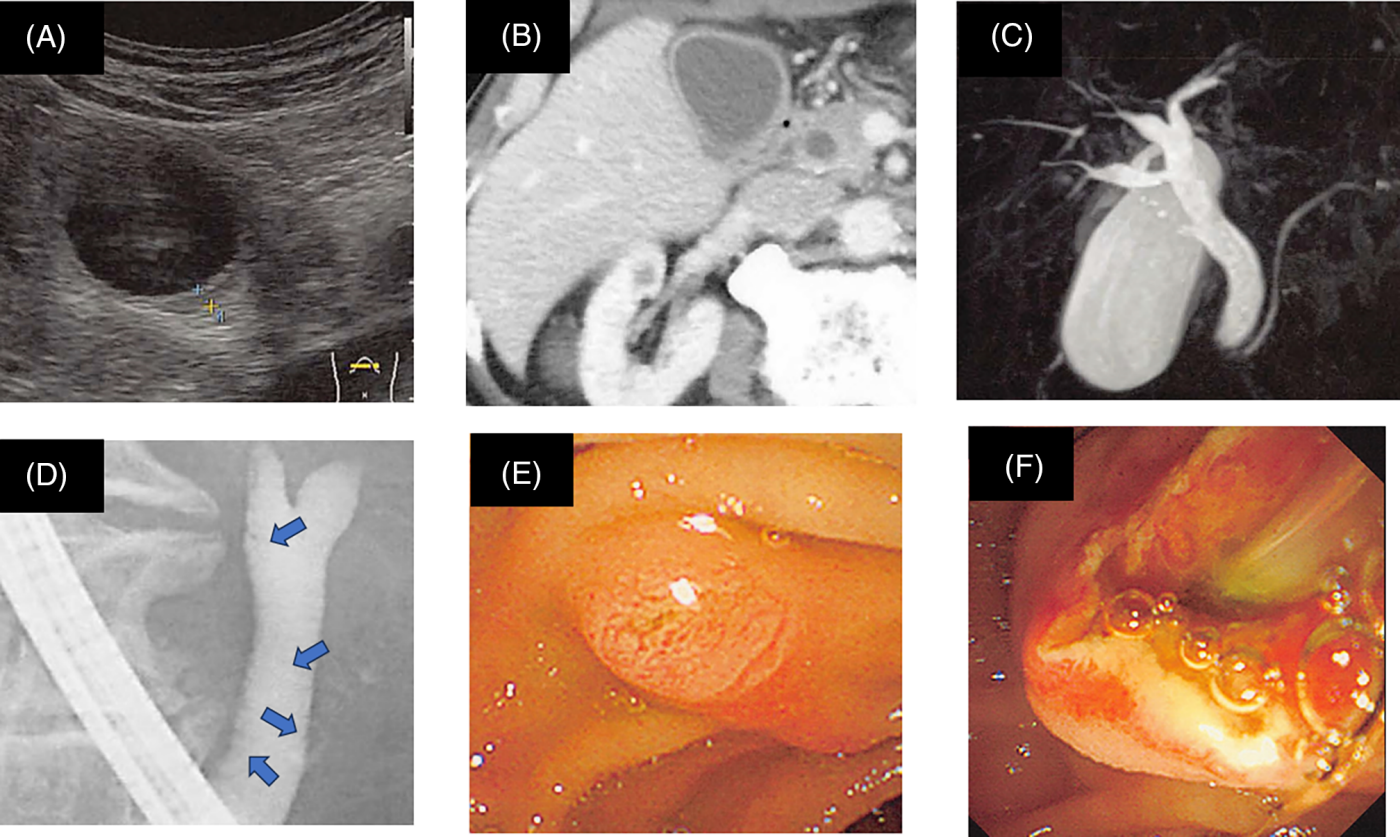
Imaging and endoscopic findings from the patient demonstrated immune‐related sclerosing cholangitis and acute obstructive suppurative cholangitis. (A) Ultrasound sonography and (B) computed tomography showed dilation of the common bile duct and thickening of gallbladder wall without stones. (C) Magnetic resonance cholangiopancreatography revealed dilation of the common bile duct and irregularly narrowed intrahepatic bile ducts. (D) Endoscopic retrograde cholangiopancreatography showed biliary sludges (indicated by arrows). (E) Endoscopic finding showed protrusion of papilla of Vater. (F) After sphincterotomy, outflow of minute biliary sands with purulent fluid was observed.
